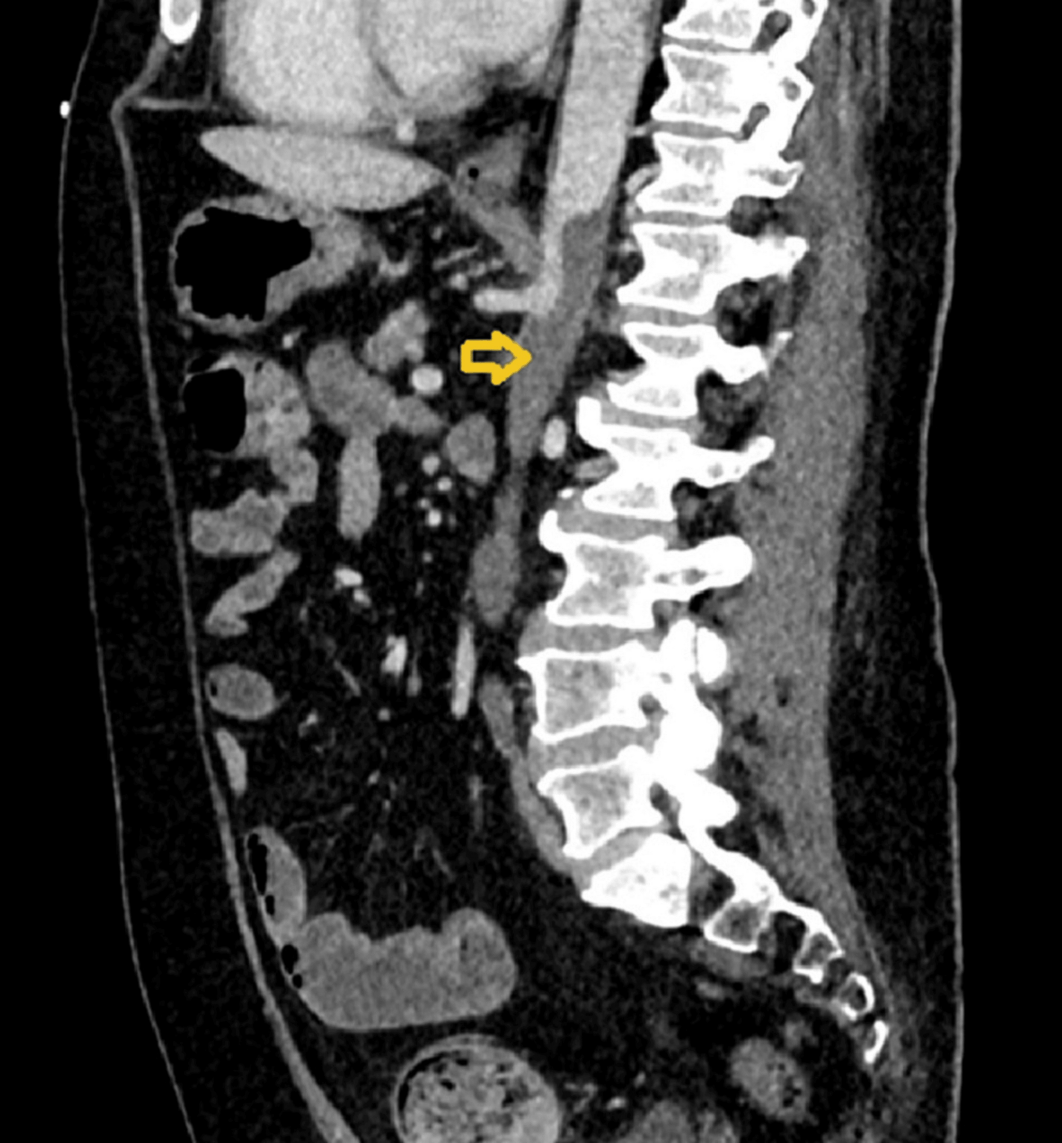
Extensive thrombosis involving multiple vascular territories Contrast-enhanced computed tomography (sagittal view) of the abdomen demonstrated extensive thrombosis involving multiple vascular territories, including the abdominal aorta distal to the coeliac axis, with thrombus extension into the internal and external iliac arteries (yellow arrow).
